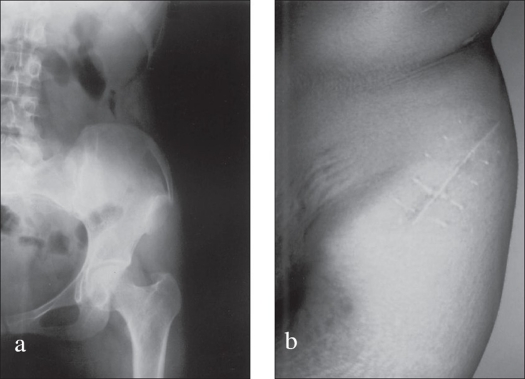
X-ray pelvis a) Showing iliac crest after incorporation of rib graft. clinical photo, b) of the same patient.
